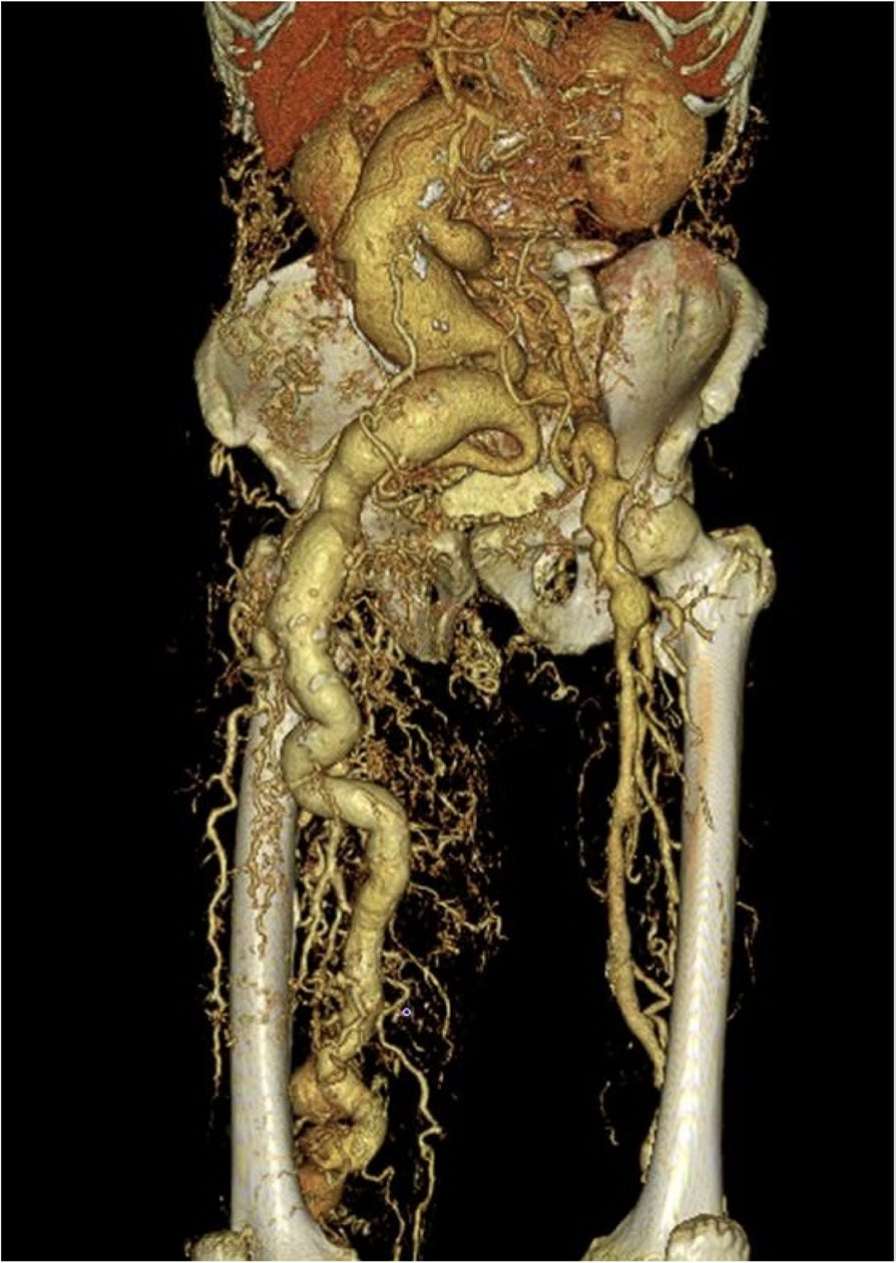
Preoperative abdominal three-dimensional computed tomography
